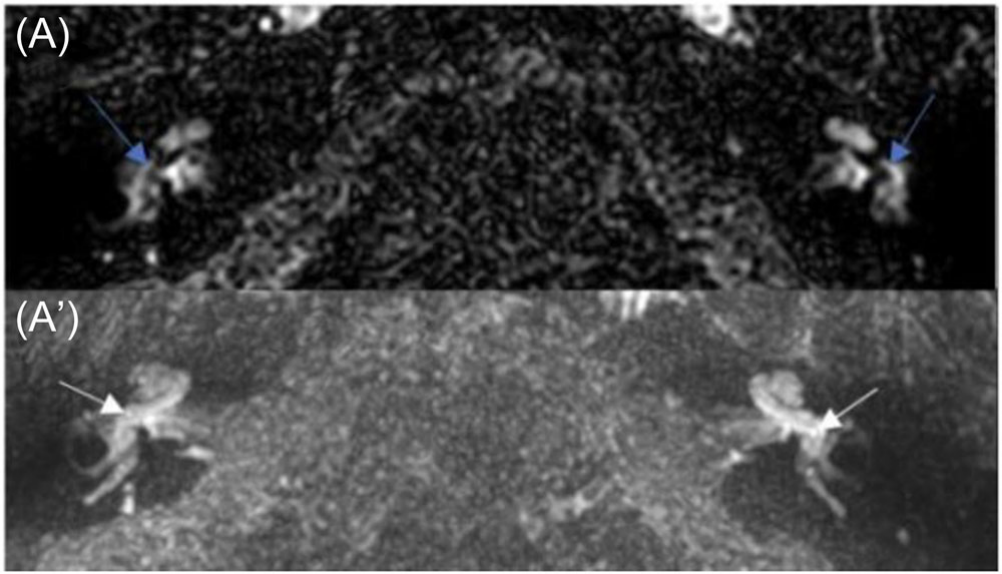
Case 3, preoperative magnetic resonance imaging. (A) The endolymphatic compartment is clearly visible and appears normal (blue arrow showing saccule). (A′) Slight blood‐labyrinth barrier impairment of the basal turn of the cochlea (white arrow).
